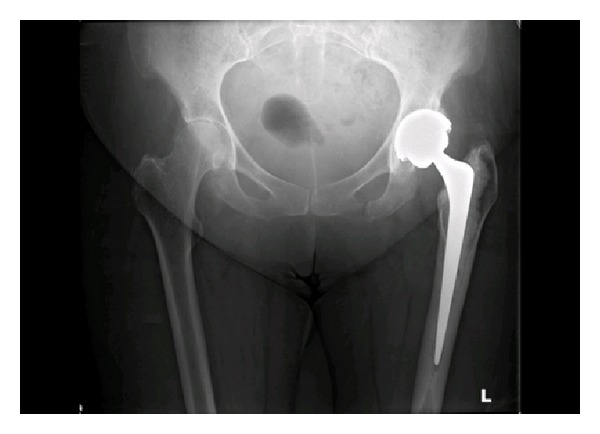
Plain radiograph taken following initial presentation with a fall, showing a fracture of the left inferior pubic ramus.
